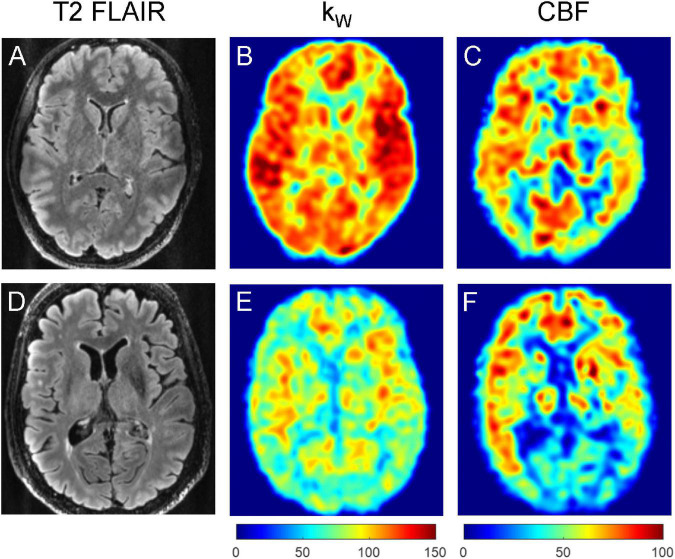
Representative T2-FLAIR images (A,D), k_W_ maps (B,E), and CBF (C,F) maps obtained from a 32-year-old subject with no vascular risk factors (A–C) and a 77-year-old subject with one vascular risk factor (former smoker, 40 pack-year) (D–F). The younger subject demonstrated mean cortical k_W_ of 108.1 min^–1^, mean white matter k_W_ of 97.4 min^–1^, mean cortical CBF of 60.1 mL/100 g/min, and mean white matter CBF of 48.6 mL/100 g/min. The older subject demonstrated lower mean cortical k_W_ of 74.8 min^–1^, mean white matter k_W_ of 70.6 min^–1^, mean cortical CBF of 44.7 mL/100 g/min, and mean white matter CBF of 37.9 mL/100 g/min.
